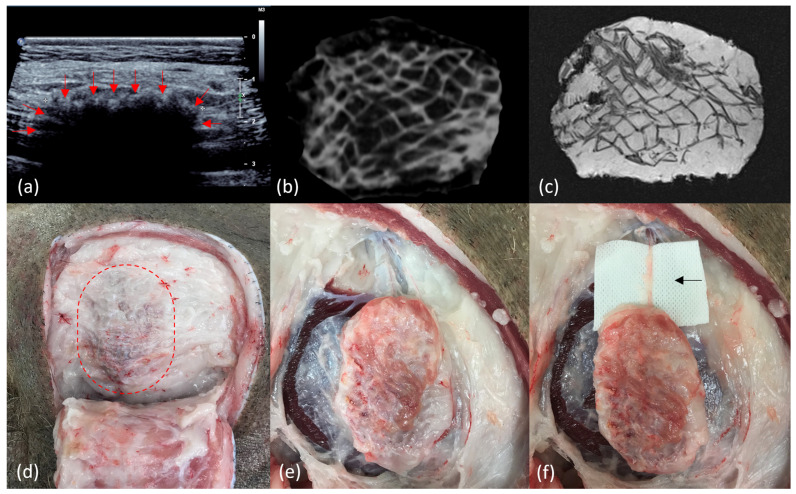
Radiological and macroscopic images of scaffolds at the 12-month end point. (a) Ultrasound of scaffold in situ, demonstrating tissue infiltration from host tissue into the scaffold marked by arrows. (b) Computed tomography of explanted scaffold demonstrating a hypodense signal of adipose tissue filling the scaffold structure, which has a hyperdense signal. (c) Magnetic resonance imaging with fat saturated (T1) sequence of explanted scaffold demonstrating the high signal of adipose tissue filling the scaffold structure, which has a low signal. (d) View of scaffold in situ (dotted line) with overlying soft tissue reflected back, demonstrating significant tissue integration and a lack of fibrous capsule formation. (e) View of scaffold partially dissected from surrounding tissue, demonstrating a lack of scaffold migration or rotation, significant tissue integration, and a lack of fibrous encapsulation. (f) View of completely dissected scaffold filled with soft tissue. There is an arrow showing a dissected blood vessel growing into the scaffold from a major blood vessel in the surrounding host tissue.
